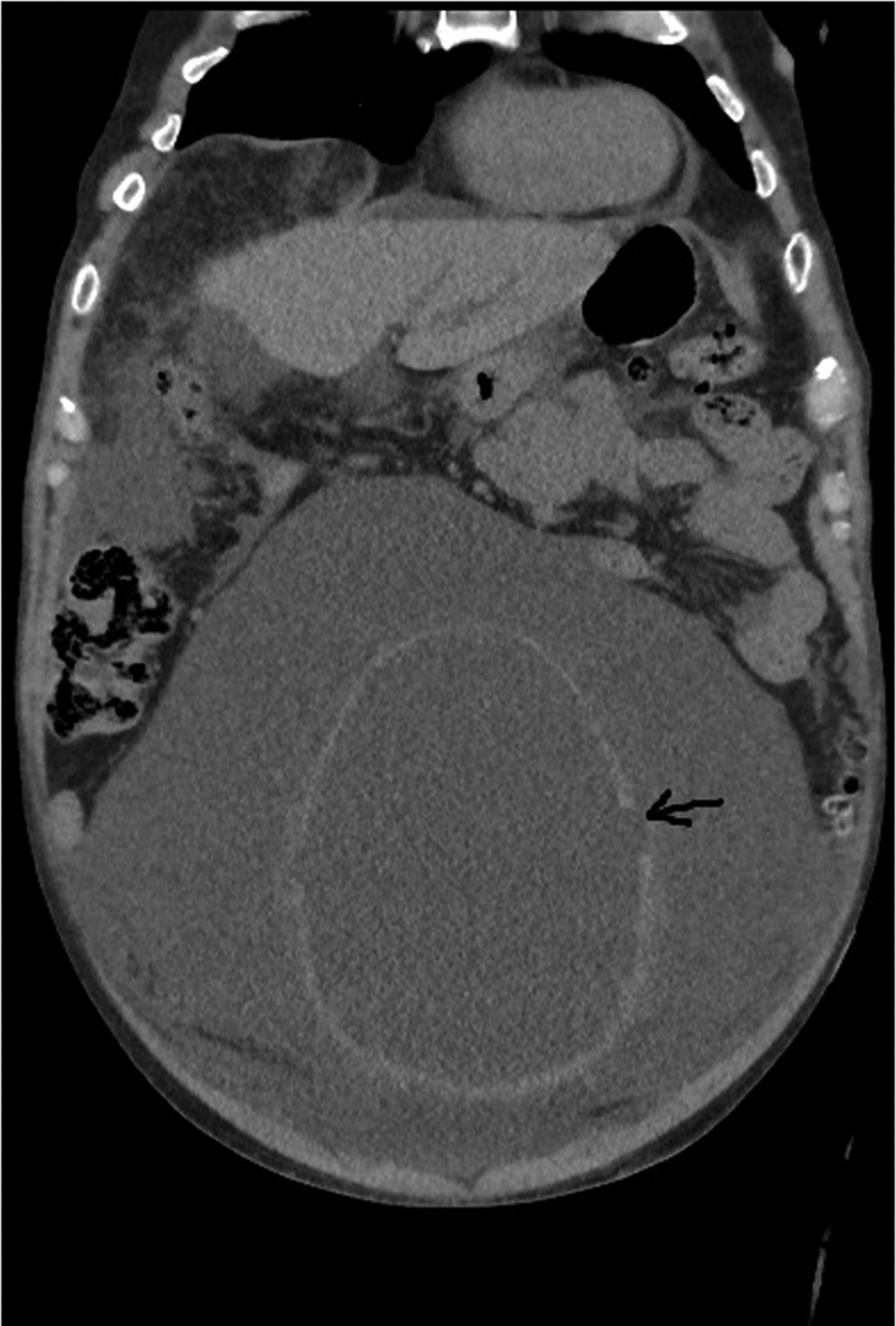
Coronal section of CT showing perforation (*arrow*) of the bladder wall with surrounding fluid collection.
